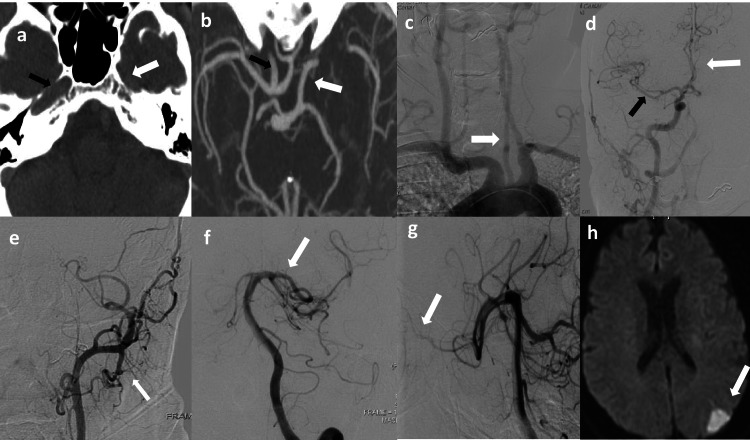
(a) CT head shows the absence of the left carotid canal (white arrow) and the well-formed right carotid canal (black arrow). (b) CT angiogram head shows basilar artery supplying left MCA through well-developed PCOM (white arrow) and right ICA supplying bilateral ACA and ipsilateral MCA (black arrow). (c) Aortic arch injection reveals a smaller caliber of the left common carotid artery (white arrow). (d) Right common carotid injection (AP view) shows right ICA suppling bilateral ACA (white arrow) and ipsilateral MCA (black arrow). (e) Left common carotid injection (AP view) reveals the absence of left ICA and opacification of ECA branches (white arrow). (f) Left vertebral artery injection (AP view) showing the well-developed PCOM (white arrow) supplying left MCA. (g) Left vertebral artery injection (Lateral view) shows the ophthalmic artery (white arrow) coming off the MCA. (h) MRI brain showing left MCA-PCA acute watershed infarct (white arrow). CT: computed tomography; MCA: middle cerebral artery; PCOM: posterior communicating artery; ICA: internal carotid artery; ACA: anterior cerebral artery; ECA: external carotid artery; MRI: magnetic resonance imaging; AP: anterior-posterior
